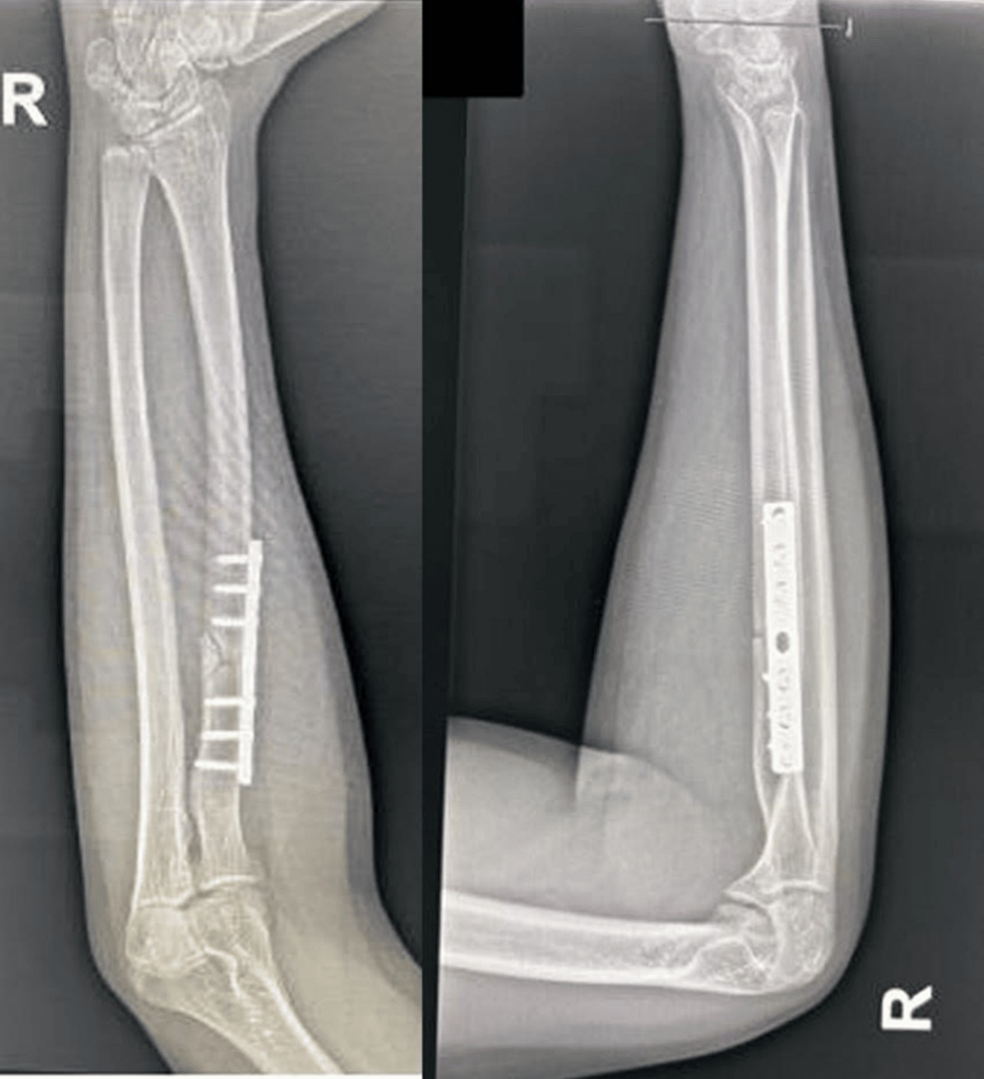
Postoperative AP and lateral view X-rays of the right forearm
